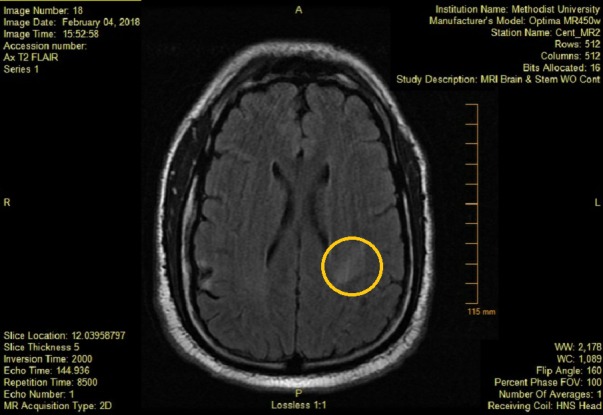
MRI FLAIR showing new area of enhancement after symptoms of stroke. Area of interest marked by circle. MRI FLAIR: Magnetic resonance imaging fluid-attenuated inversion recovery.
